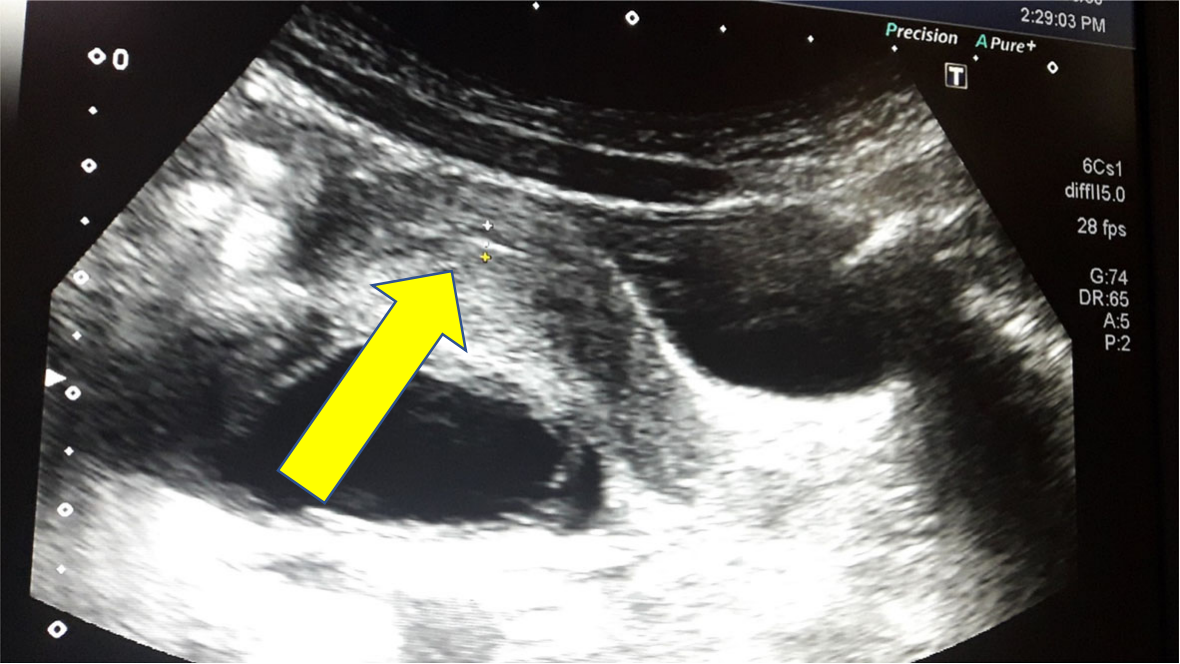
Ultrasound scan image of enlarged uterus (yellow arrow) measuring 3.6 cm × 1.9 cm × 2.5 cm and endometrial thickness of 4 mm
